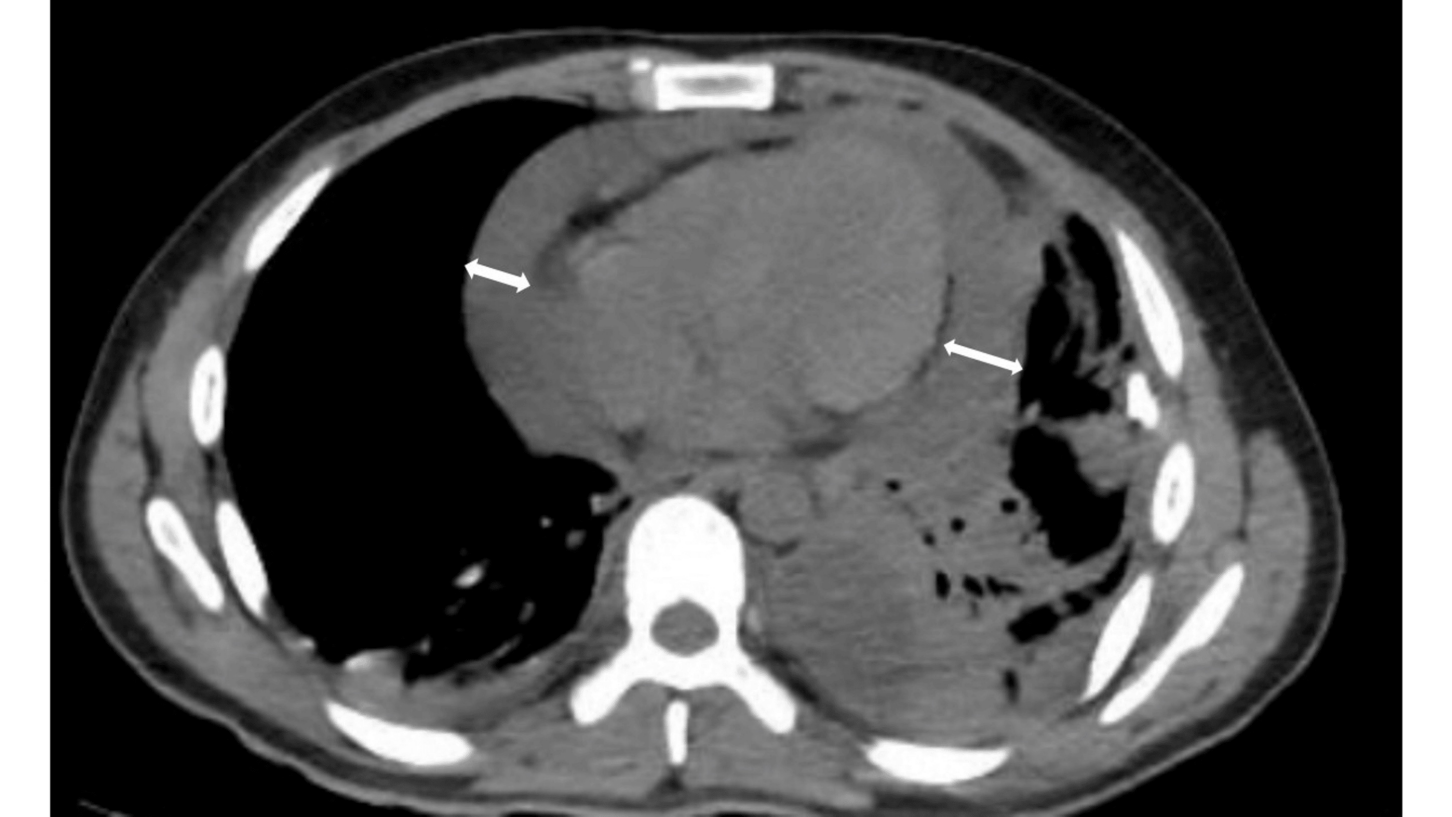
Repeat chest CT on day 10 showing a newly developed, large pericardial effusion. This imaging was obtained after completion of intrapleural fibrinolytic therapy and coincided with a significant drop in hemoglobin and worsening shortness of breath. The pericardial effusion prompted surgical intervention with evacuation of 500 mL of hemorrhagic fluid.
